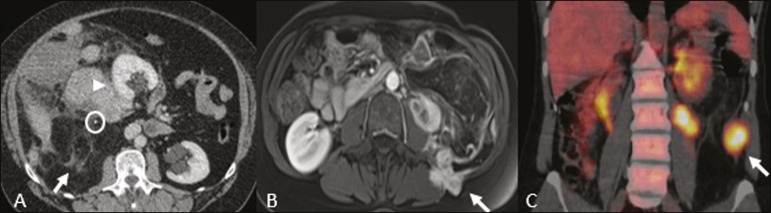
Dedifferentiated liposarcoma. A: 58-year-old male. CT
